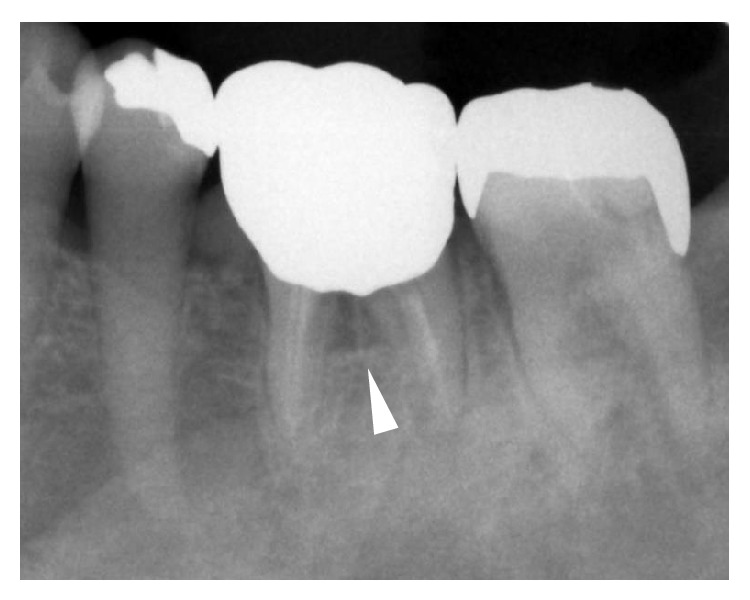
Dental X-ray indicated apparent furcation involvement of the left mandibular first molar.
